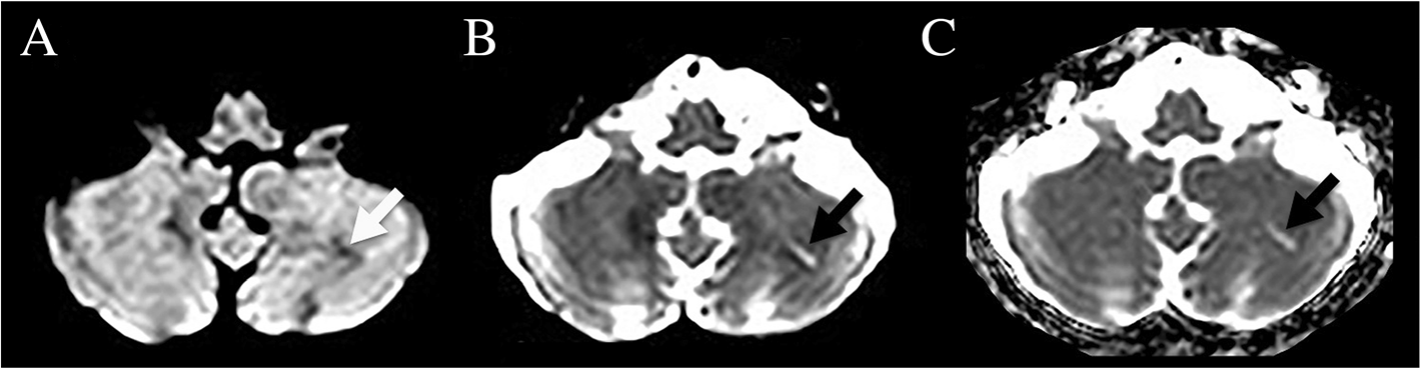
MRI showing a patient with chronic SCCI, seen as hypointensity on DWI B1000 (a) and as hyperintensity on B0 images (b) and ADC map (c)
